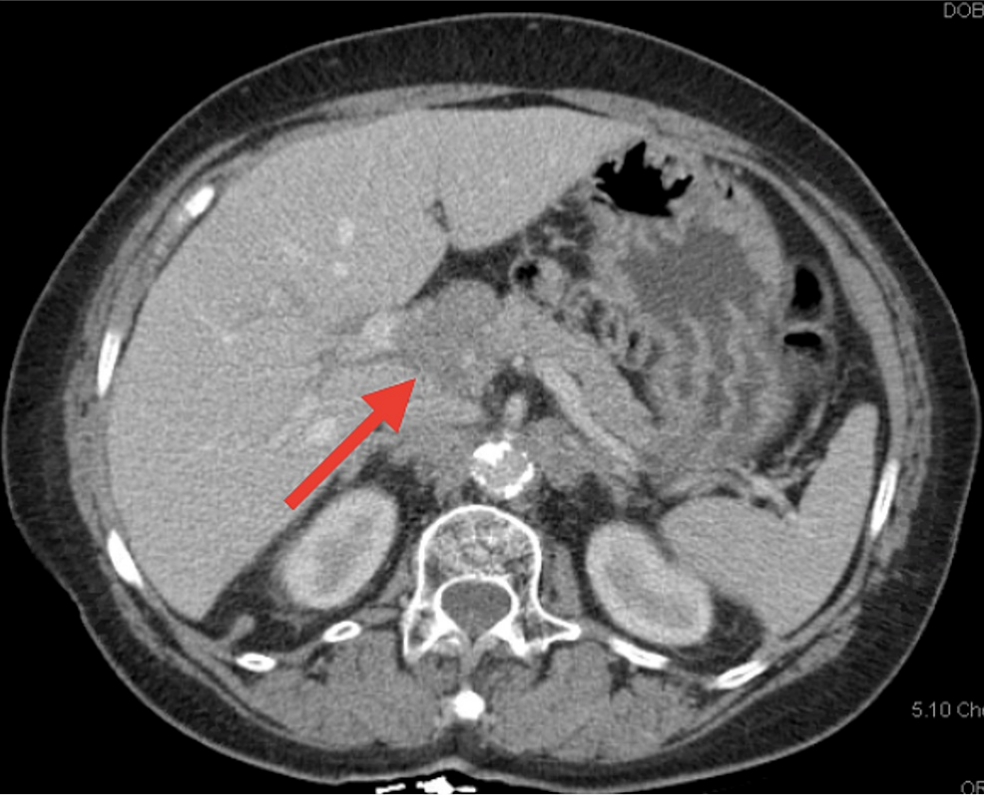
Spiral abdomen and pelvic CT demonstrating enlarging metastatic lymphadenopathy within gastrohepatic ligament shown with a red arrow.
